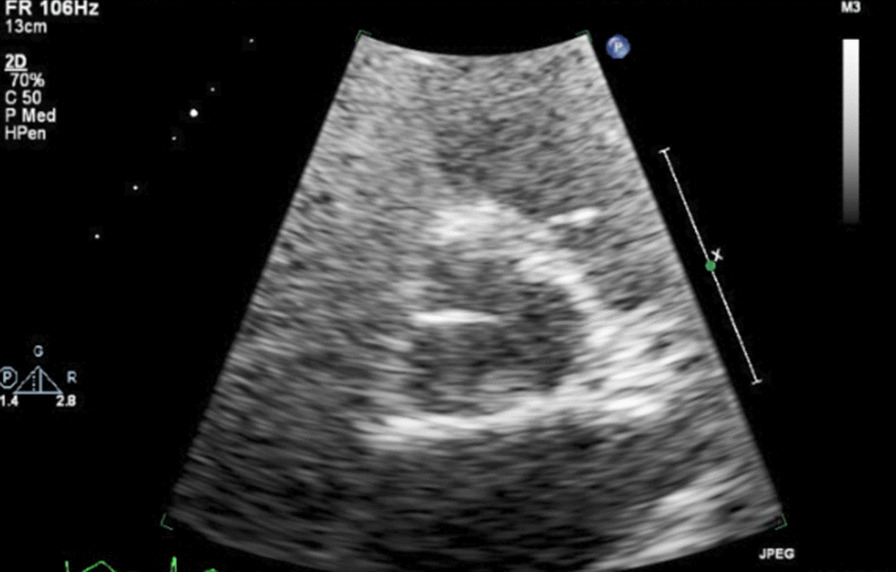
Transthoracic echocardiogram in parasternal short axis view showing aortic valve annular calcification
